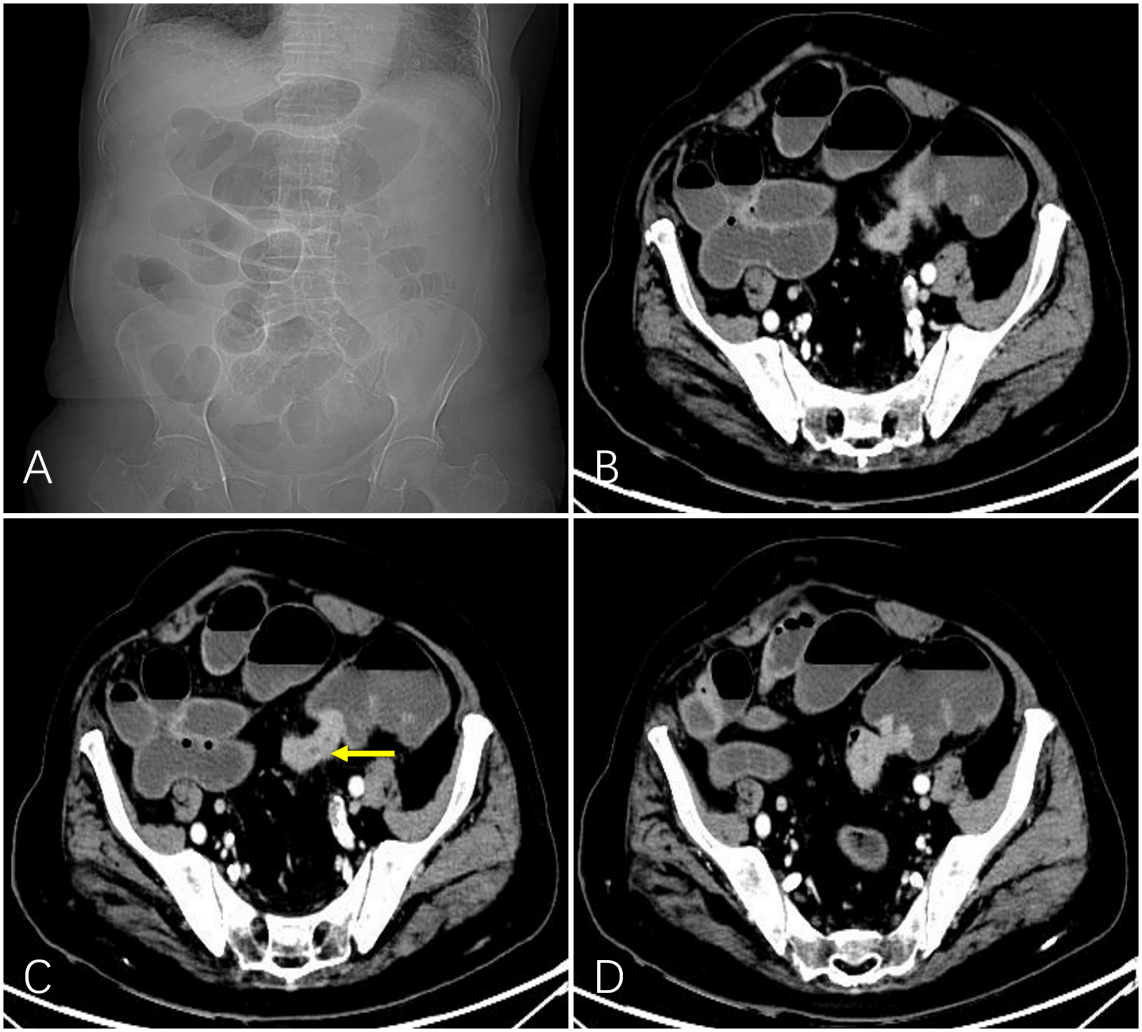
A 92-year-old man with obstructed bowel movements for 10 days and worsening for 3 days. Abdominal plain film indicated intestinal gas and fluid accumulation (A). Preoperative computed tomography showed marked localized thickening of the sigmoid colon wall (indicated by the yellow arrow) and enhancement and corresponding narrowing of the intestinal lumen. The upstream intestinal tract was significantly dilated due to air and fluid accumulation (B-D).
